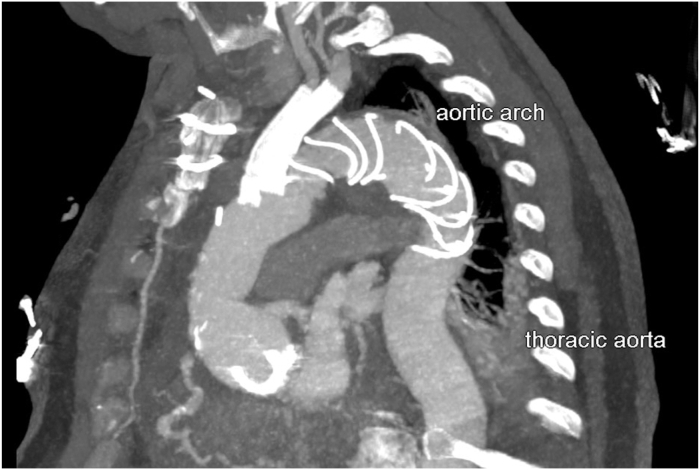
Postoperative contrast-enhanced computed tomography angiography in sagittal view.
